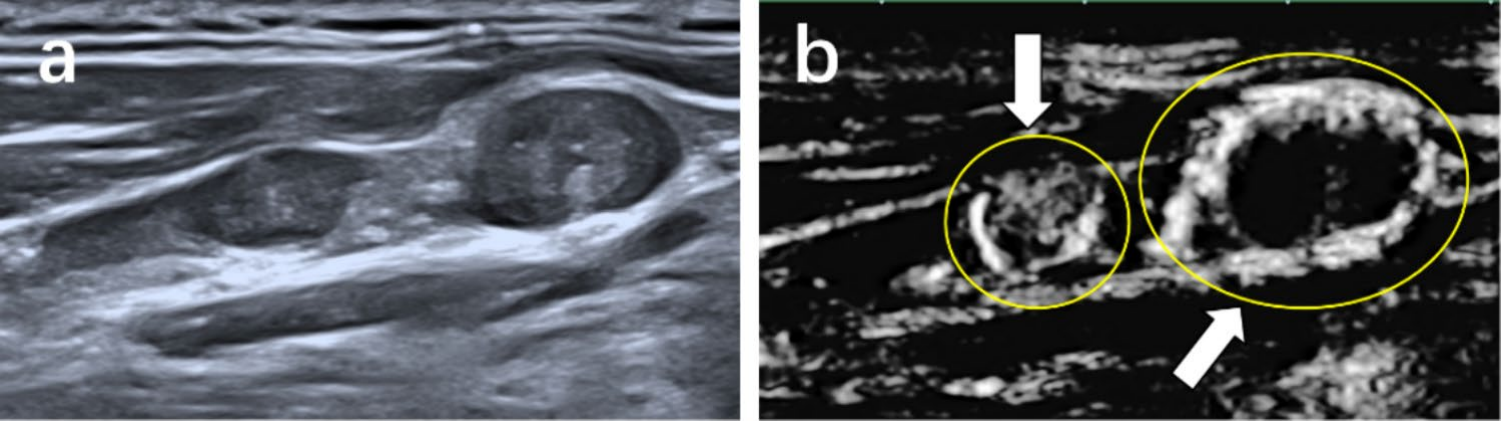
Metastatic lymph node from a 39-year-old man. a Conventional ultrasound. b Superb microvascular imaging (SMI), the lymph node exhibits ring-shaped vessel distribution
